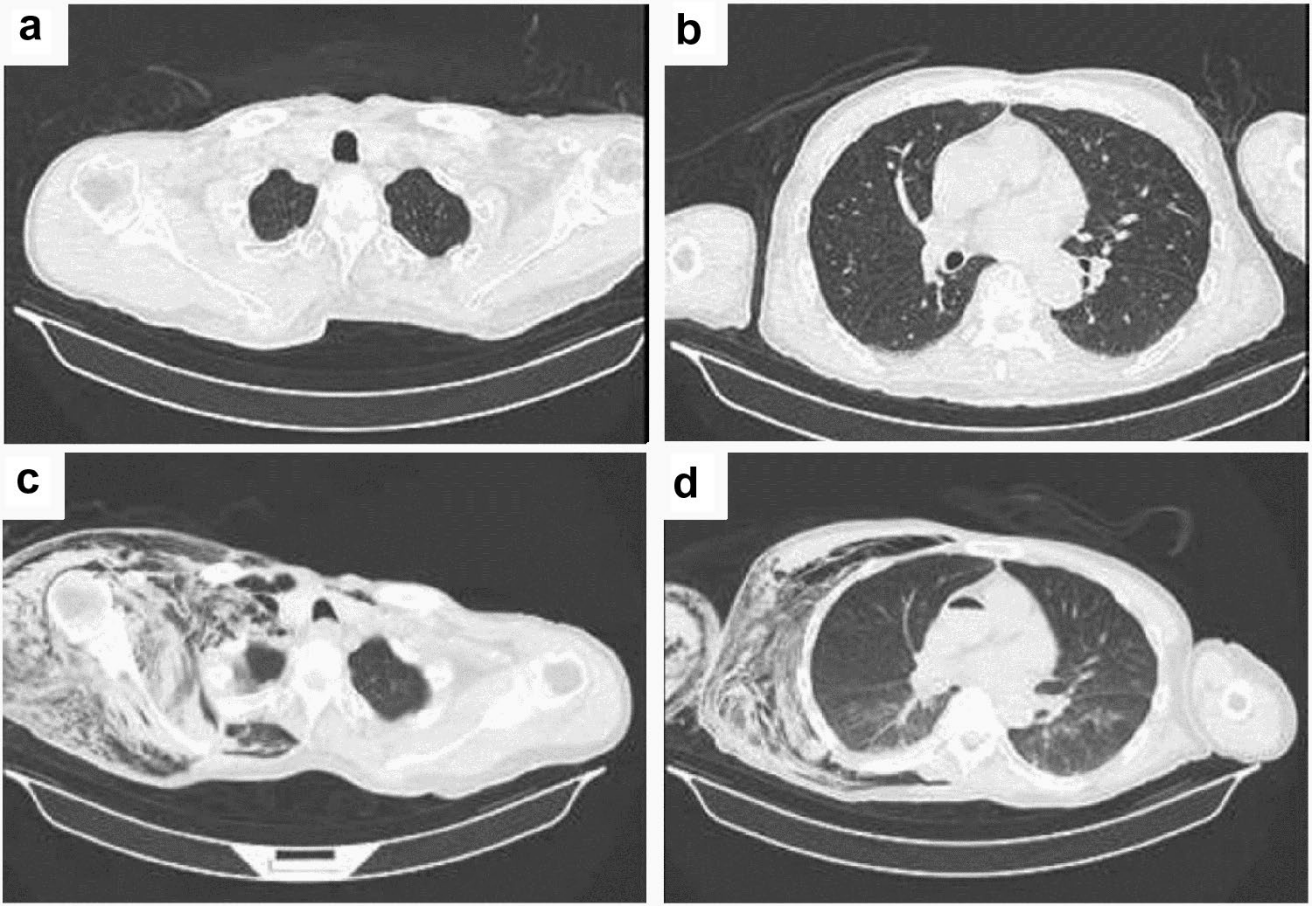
Chest computed tomography images on admission (upper panels) and postmortem computed tomography images (lower panels): a no remarkable changes were observed in subcutaneous regions and muscles. b No remarkable changes were observed in lung and cardiovascular system. c A massive amount of gas was observed in the right upper extremity. d Accumulation of gas was observed both within the torso and in the aorta
